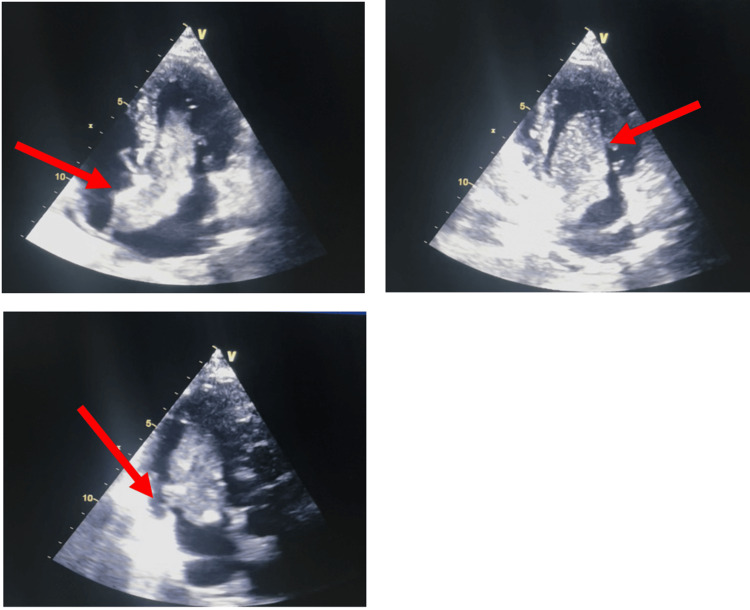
Transthoracic echocardiographic views (apical four-, two-, and three-chamber views showing a large, mobile heterogeneous mass in the left atrium, attached to the interatrial septum and prolapsing into the mitral valve during diastole. Top left (four-chamber view): A large, heterogeneous mass is seen arising from the interatrial septum, prolapsing into the left ventricle through the mitral valve during diastole. Top right (two-chamber view): The mass is clearly visualized within the left atrium, extending toward the mitral orifice, highlighting its mobility and risk of obstruction. Bottom (three-chamber view): The lesion appears heterogeneous and mobile, protruding into the left ventricular inflow tract, further supporting the diagnosis of a myxoma.
